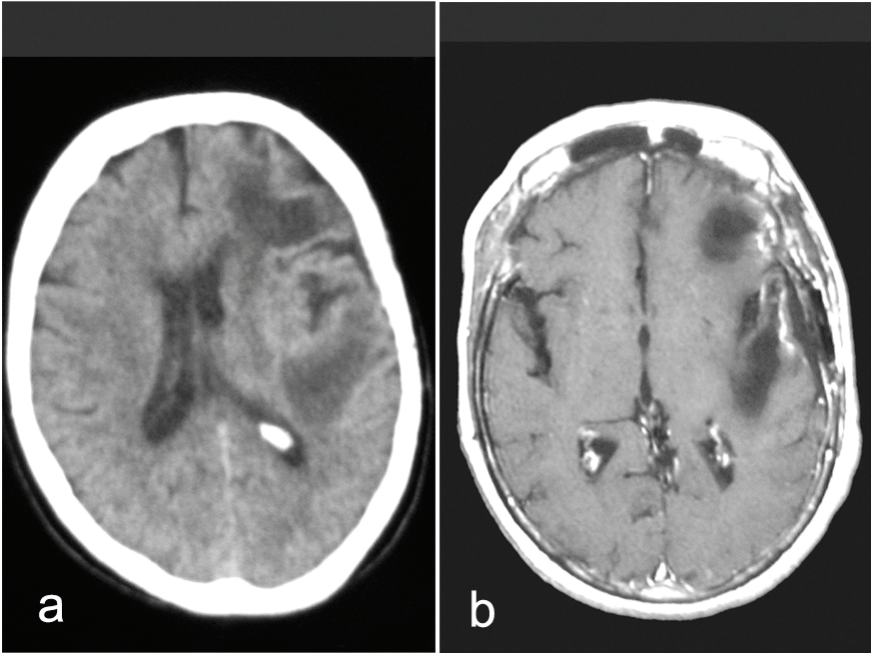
In postoperative CT (a) and MR (b) images were observed that both cystic masses got smaller.
